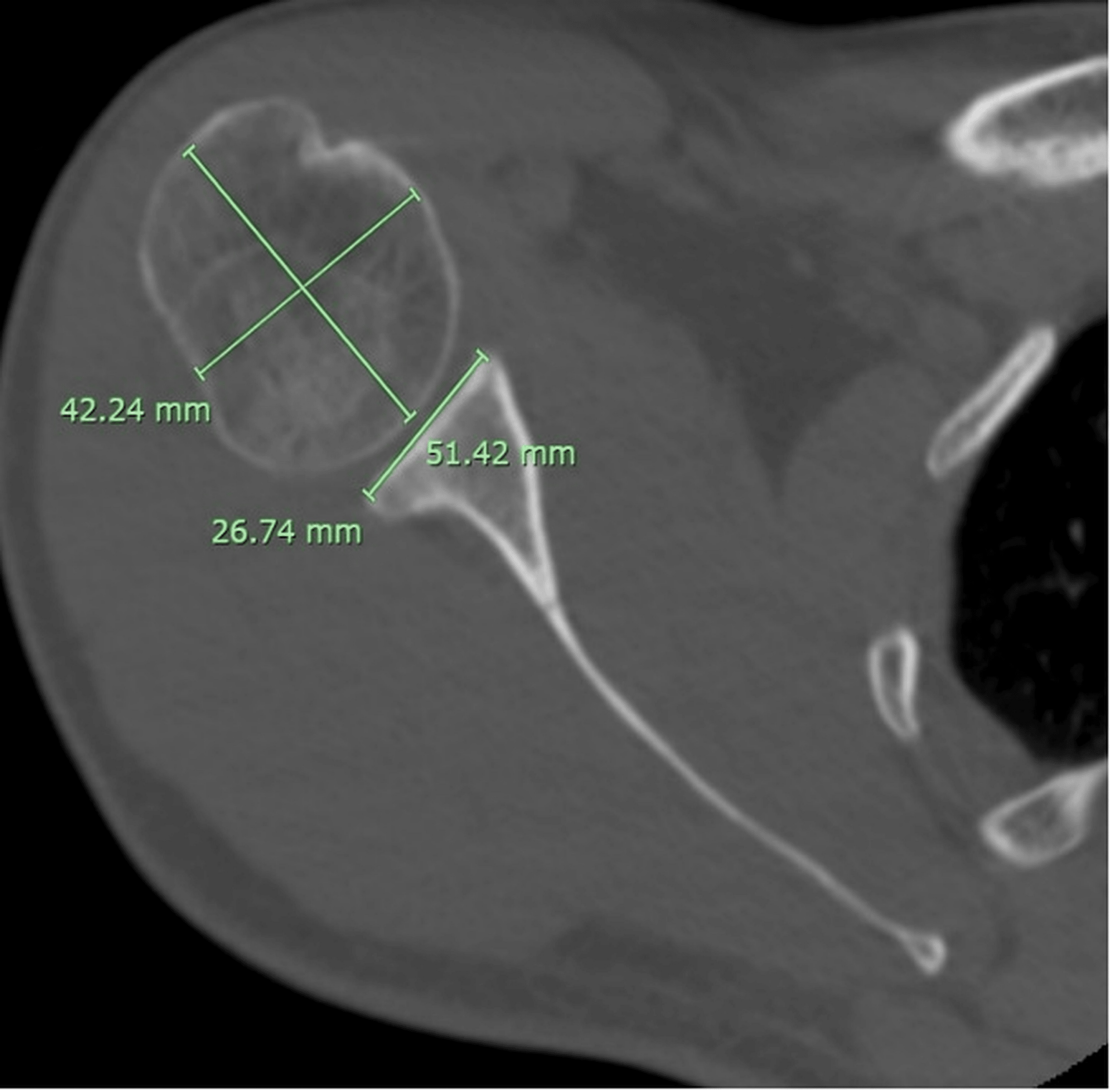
Measurement of the humeral head diameter on axial CT imaging
